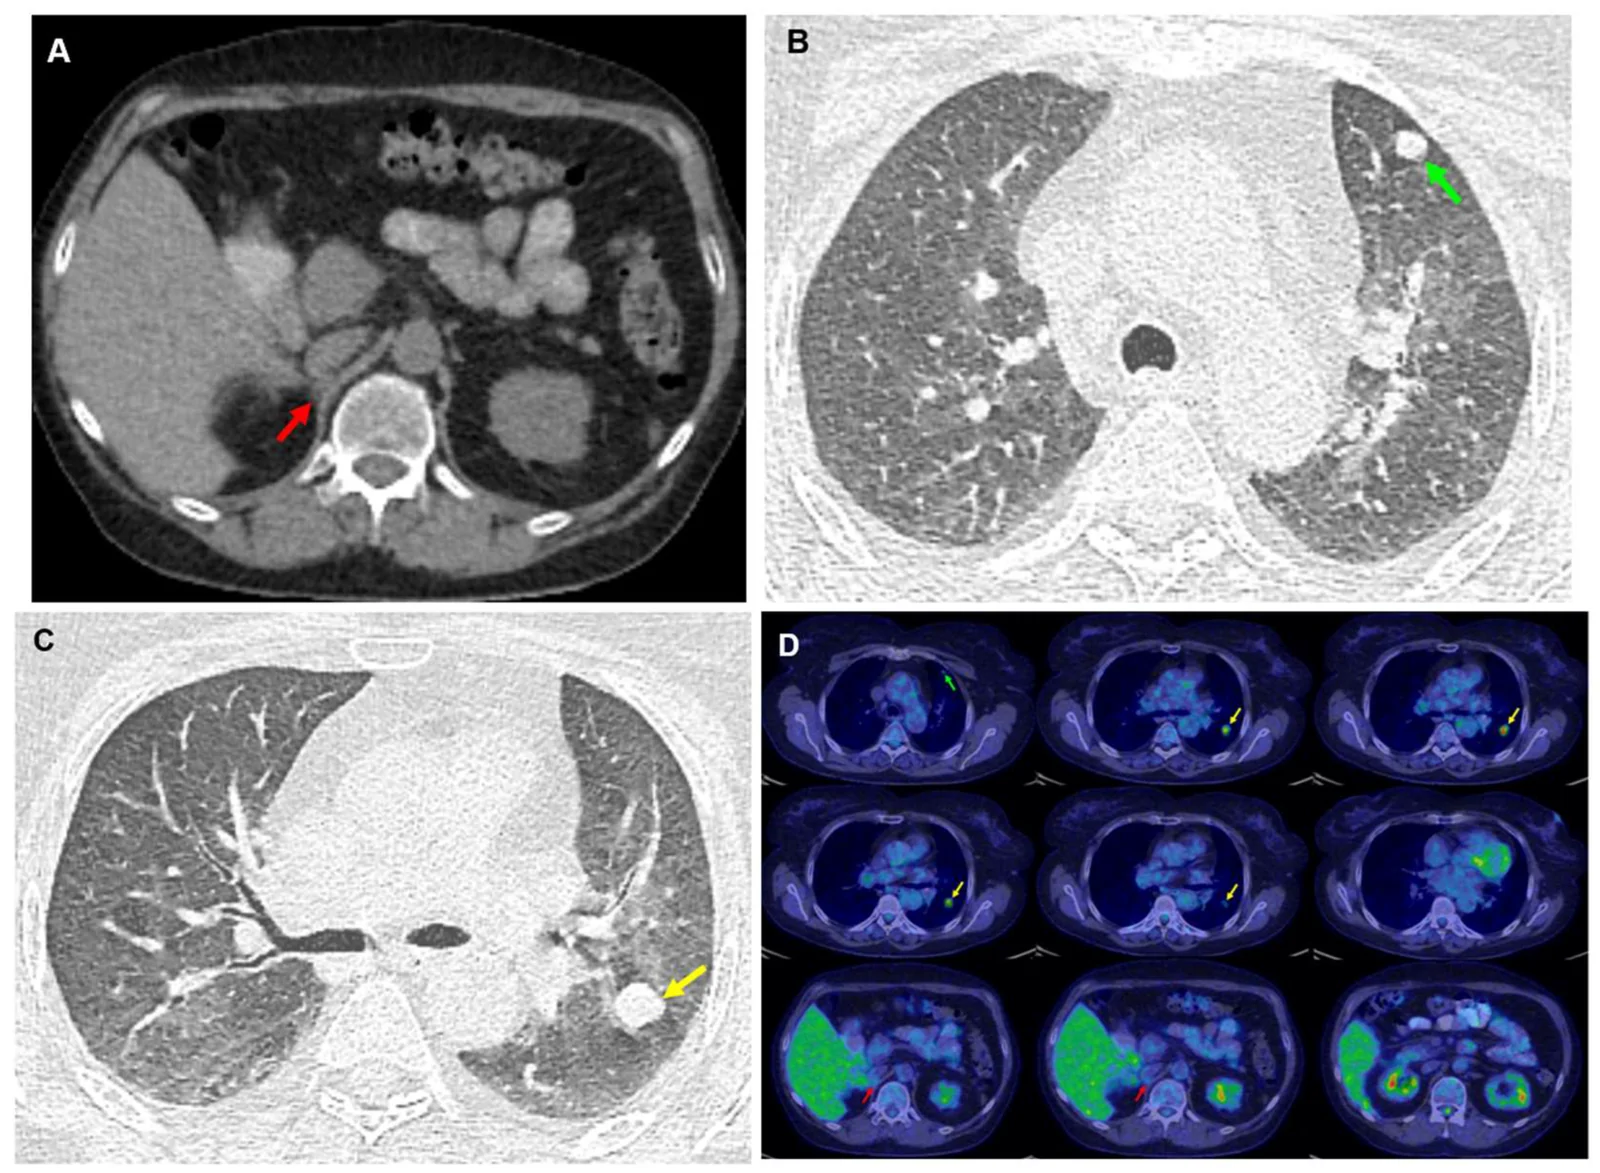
In accordance with the patient’s option for mitotane withdrawal, she remained under endocrine surveillance as an outpatient for another four years (between 2018 and 2020), while she felt well and continued all the mentioned replacement hormonal therapy for the adrenal and thyroid insufficiency [CT scan in the year 2018 (A–C): (A) right oval retrocaval mass (red arrow) of 8 by 7.5 by 11 mm indicating ACC recurrence (native CT scan, axial plane); (B) pulmonary nodule of 8 mm (green arrow) in the anterior segment of the left upper lobe (native CT scan, axial plane, lung window); (C) pulmonary nodule of 14 mm (yellow arrow) in the apico-posterior segment of the left upper lobe (native CT scan, axial plane, lung window); positron emission tomography (PET-CT, axial plane) in the year 2018 (D): pulmonary nodule of 8 mm (green arrow) in the anterior segment of the left upper lobe without focal metabolic activity; metabolically active pulmonary nodule of 14 mm (yellow arrow) in the apico-posterior segment of the left upper lobe; metabolically active right oval retrocaval mass of 8 by 7.5 by 11 mm (red arrow) suggestive of a local ACC recurrence].
